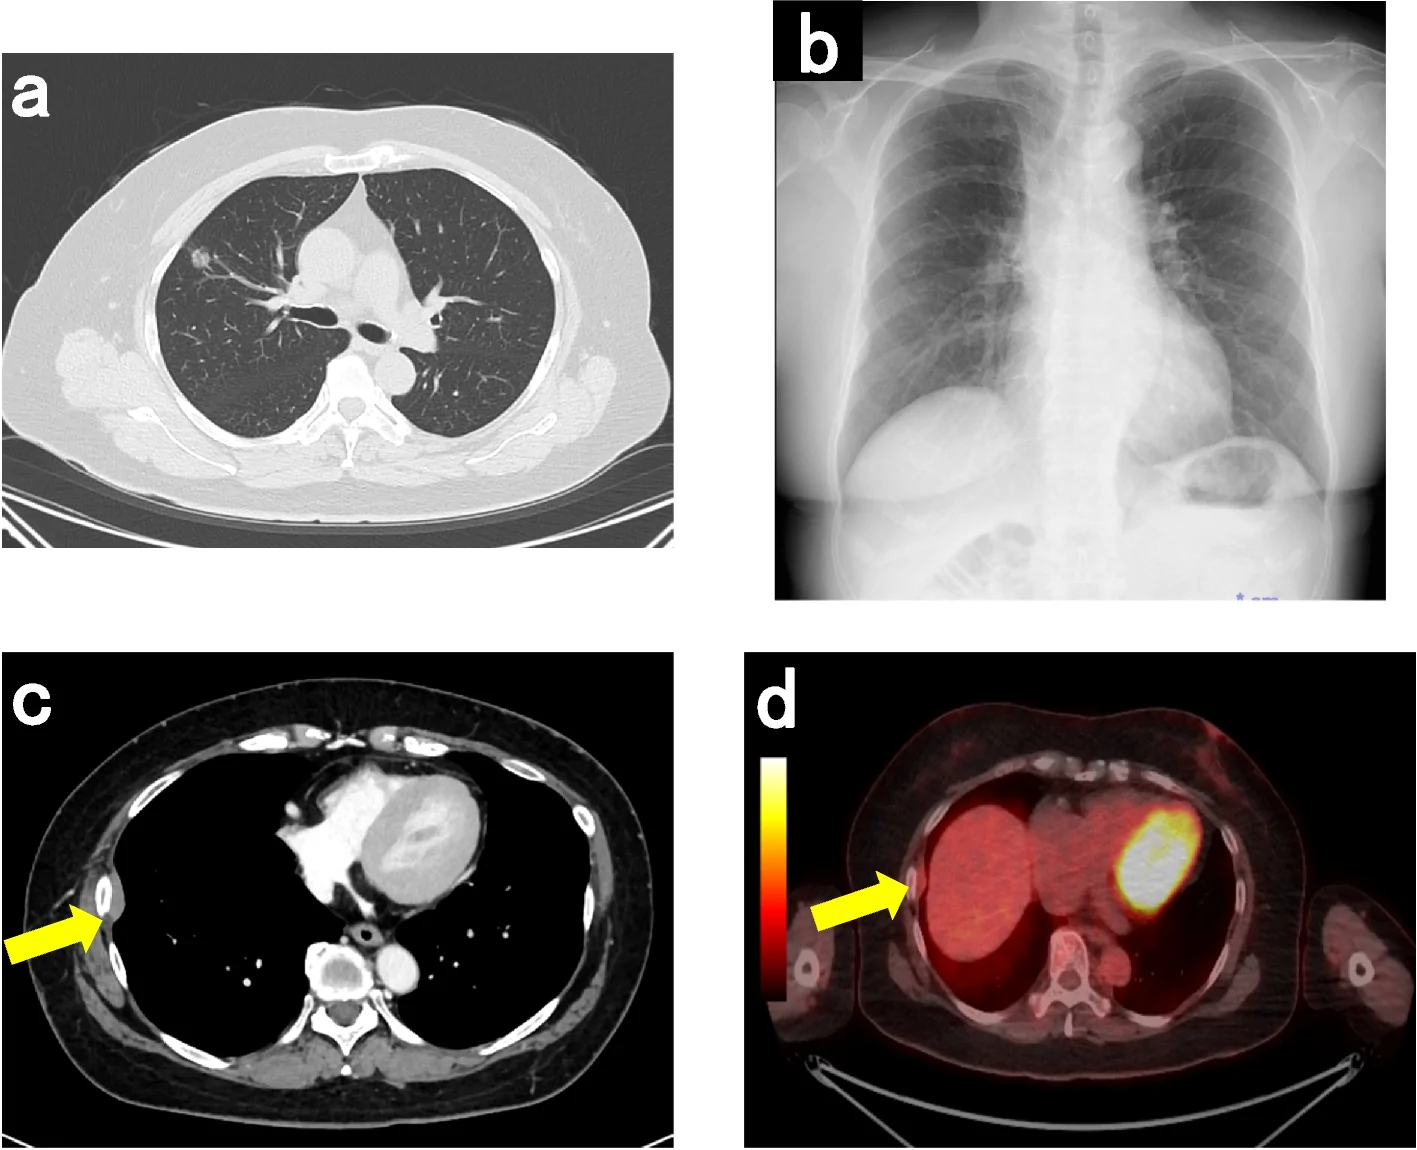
Imaging findings of the primary lung cancer and the postoperative port-site lesion. a Preoperative chest computed tomography demonstrating the primary lung adenocarcinoma. b Upright chest radiograph demonstrating a chest wall mass abutting the pleura at a previous thoracoscopic port site. c Contrast-enhanced computed tomography demonstrating a chest wall mass adjacent to the thoracoscopic port site, abutting the pleura. d Positron emission tomography/computed tomography with fluorine-18 fluorodeoxyglucose showing mild uptake in the lesion (maximum standardized uptake value, 2.5)
